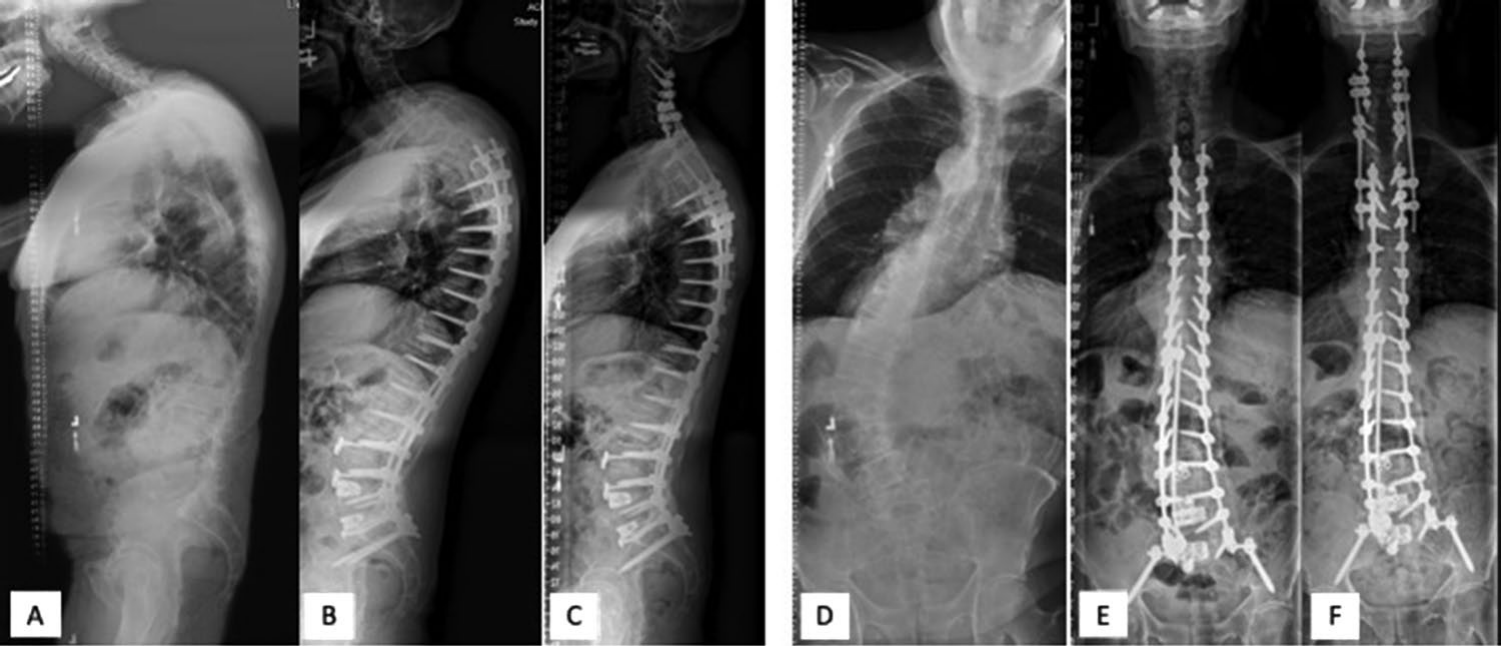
Representative radiographic example of a patient who underwent C2-pelvis posterior instrumented fusion for adult spinal deformity. A 67-year-old male with concomitant thoracolumbar scoliosis and cervicothoracic kyphosis (A, D) who was treated in a staged approach. First, he underwent an L3–S1 anterior lumbar interbody fusion and T3-pelvis posterior instrumented fusion (B, E) to address the thoracolumbar scoliosis. Three months later, an extension of posterior instrumentation to C2 with C7–T4 posterior column osteotomies was performed to address the persistent cervicothoracic deformity (C, F)
